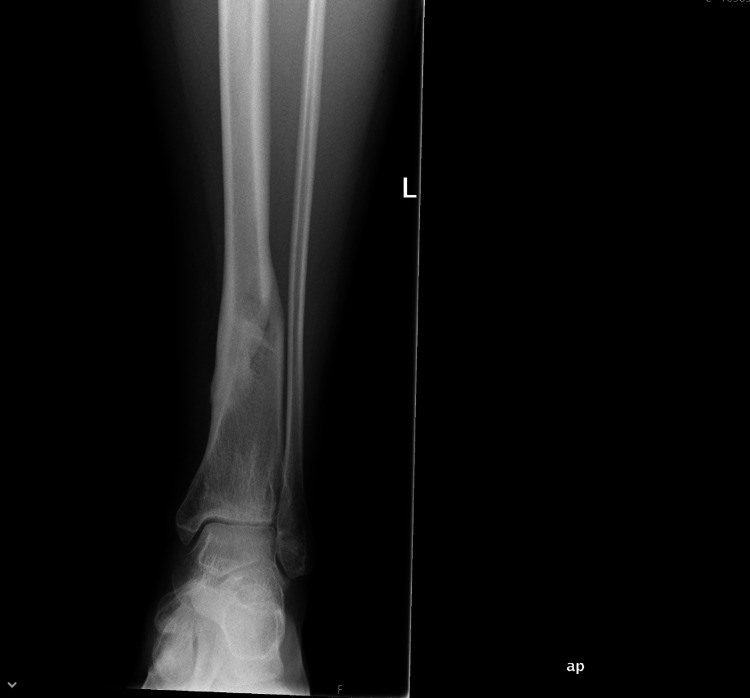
Union status at the completion of 6 months of LIPUS therapy (AP View) AP - Anteroposterior; LIPUS - Low-Intensity Pulsed Ultrasound
